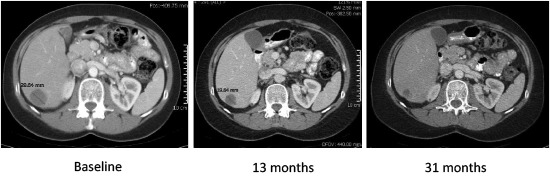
Serial CT images from a subject who achieved a sustained PR response on nelfinavir in target lesion in liver
